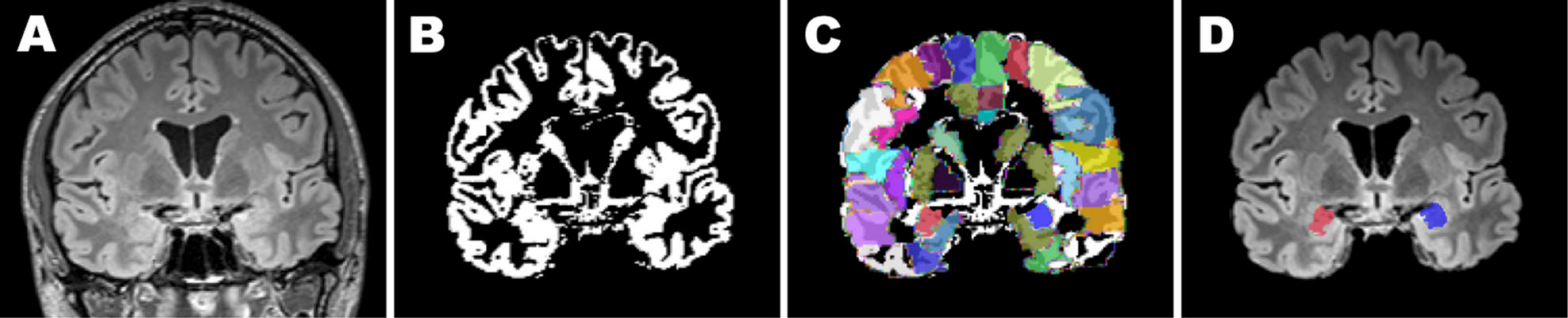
Example of automated warp-based segmentation of amygdala volumes. T2-weighted 3D-FLAIR datasets of the brain (A) were used for automated brain extraction (B). The obtained transformation matrix was inverted and warped onto the Automatic Anatomical Labeling atlas (C). The total volume of white matter, gray matter and cerebrospinal fluid, as well as the amygdala volume, was calculated. (D) Right amygdala marked in red, left amygdala marked in blue
